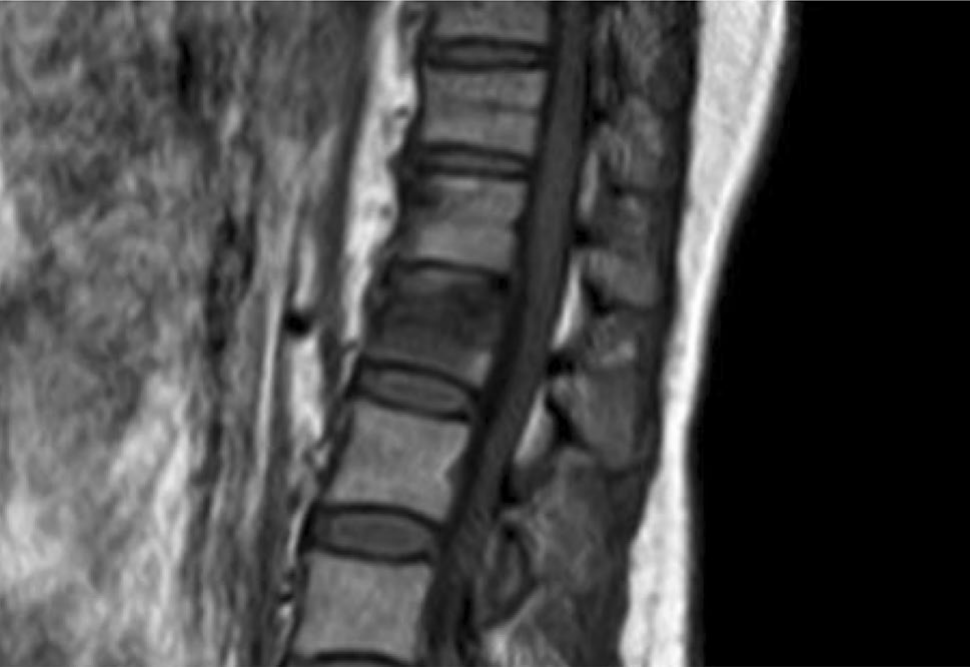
Wedge fracture*, MRI, sagittal view*
